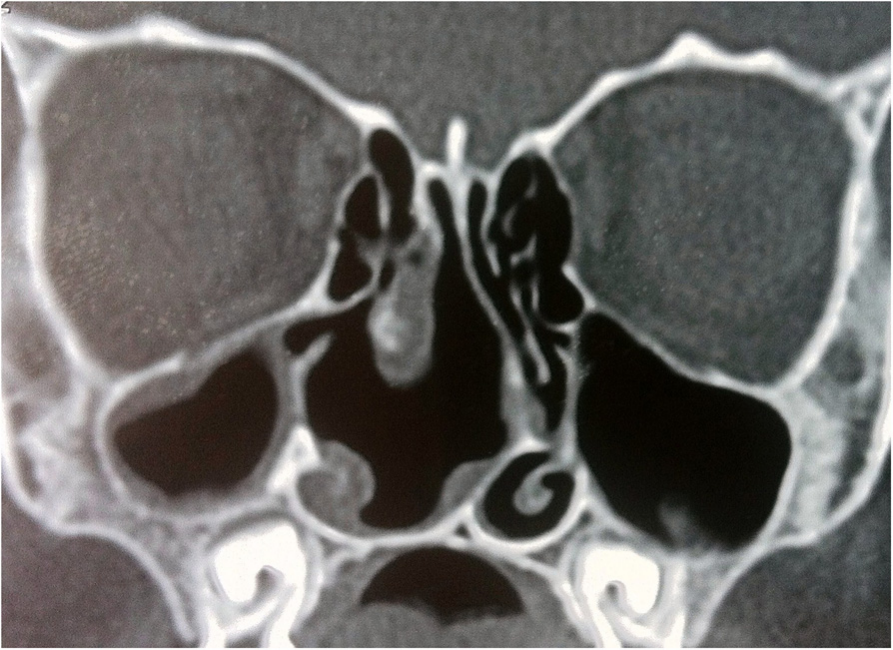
Coronal paranasal sinus computed tomography images 2 months after the operation showing no signs of recurrence or inflammation.
